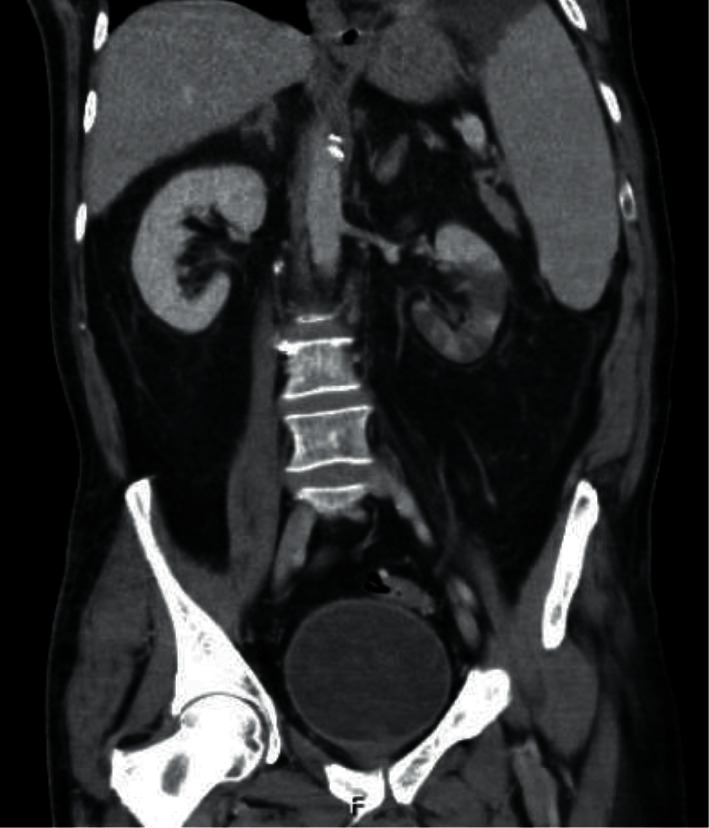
Coronal view of CT abdomen and pelvis showing numerous hypodensities of the interpolar region and lower pole of the left kidney consistent with infarctions.
